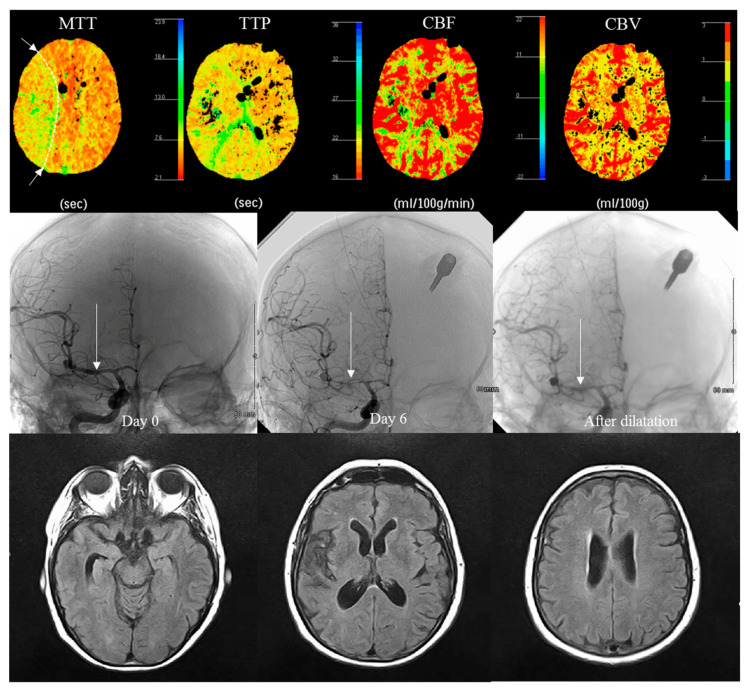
Vasospasm and its effect on PCT color maps for a given patient, 6 days after SAH with asymptomatic vasospasm (white arrow) in the right middle cerebral artery and an area of low perfusion in the territory of this artery (highlighted by a dotted line in MTT). Vasospasm detected on systematic CT (no abnormality found on TCD, no intra-cranial hypertension). No DCI was found on MRI at 3 months (the hypointensity on FLAIR in the Sylvian fissure is related to the resorption of hematoma).
